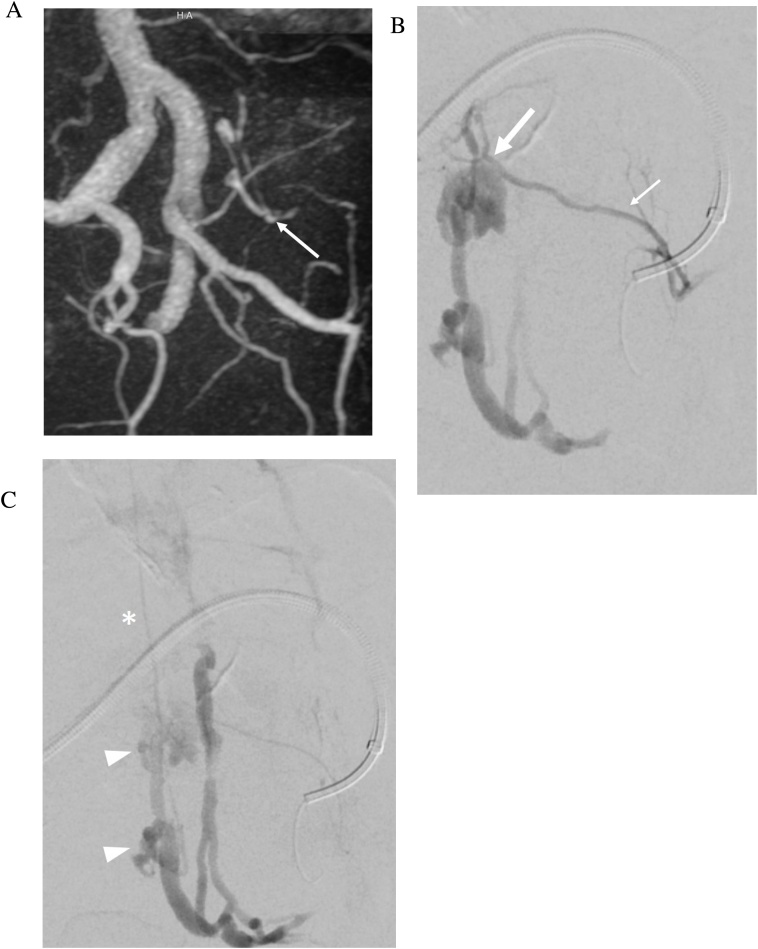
a Spinal dural arteriovenous fistula. 74-year-old man presenting with 5 months history of bilateral lower extremity weakness, numbness, and low back pain. Initial catheter spinal angiogram was negative for dural arterial venous fistula. Sagittal T2-weighted FSE MRI image (a) demonstrates central cord signal abnormality within the conus. Faint linear low signal is seen along the ventral aspect of the cauda equina (arrows). Sagittal FIESTA-C MRI image (b) clearly demonstrates a tortuous and enlarged filum terminale vein (arrows). Axial FIESTA-C MRI images (c–d) demonstrate tracking of the enlarged filum terminale vein into an engorged vessel within the left ventral epidural space at L5 and S1 (arrowheads). b Spinal dural arteriovenous fistula. 3D reconstruction of dynamic contrast enhanced MRA image (a) confirms a connection (arrow) between a branch of the left internal iliac artery (iliolumbar artery) and the abnormal vessel within the left L5 and S1 ventral epidural space. Digital subtraction angiogram images (b–c) shows selective contrast injection into the left iliolumbar artery (thin arrow) with demonstration of the fistula at the level of the L5-S1 neural foramen (thick arrow). Venous drainage is to dilated arterialized ventral epidural varices at L5 (arrowheads) from which an ascending filum vein is noted to fill (asterisk)..
